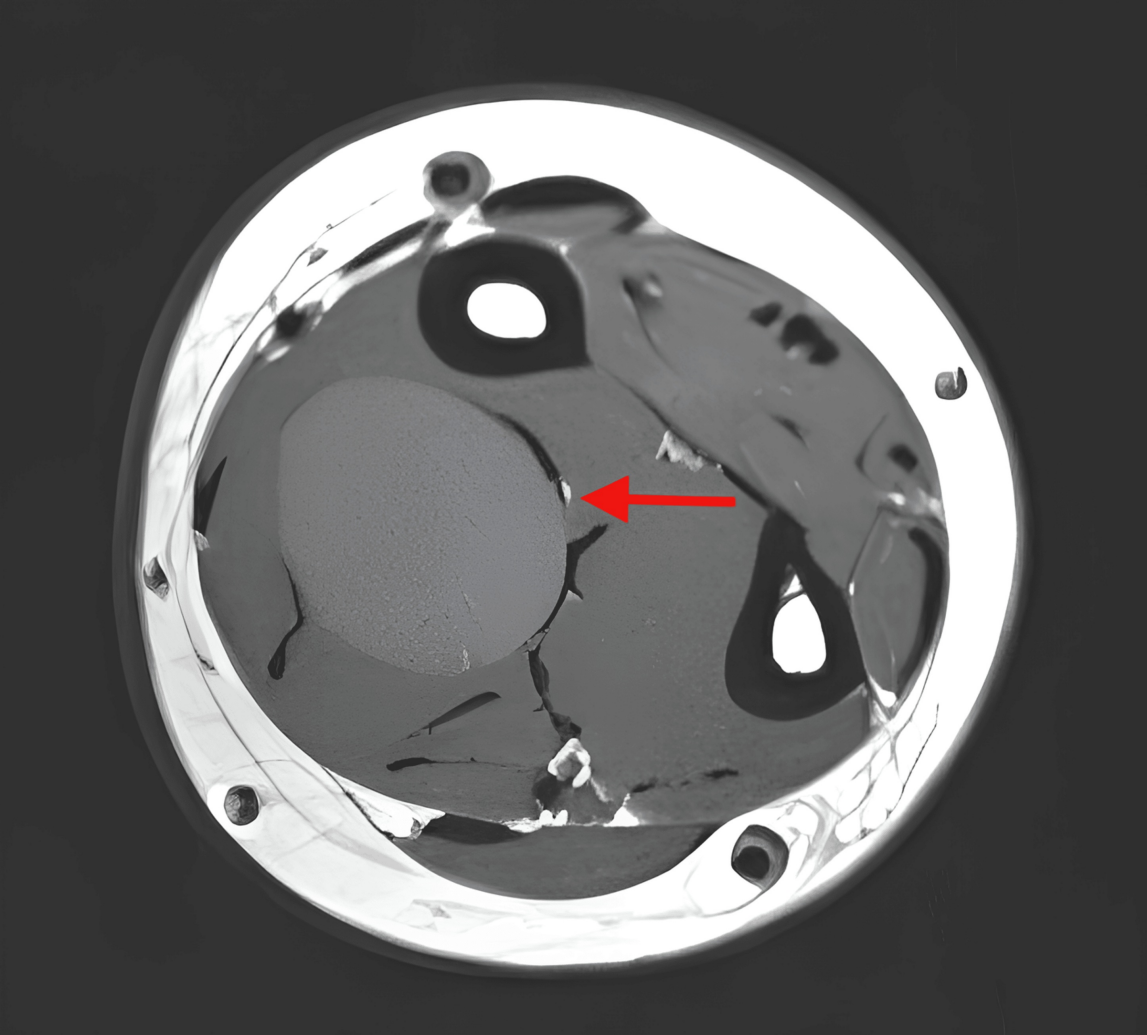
T2-weighted axial MRI image of the right forearm The red arrow shows a well-defined, oval-shaped heterogeneous hyperintense lesion in the volar compartment of the right forearm, with no infiltration into the adjacent tissues.
